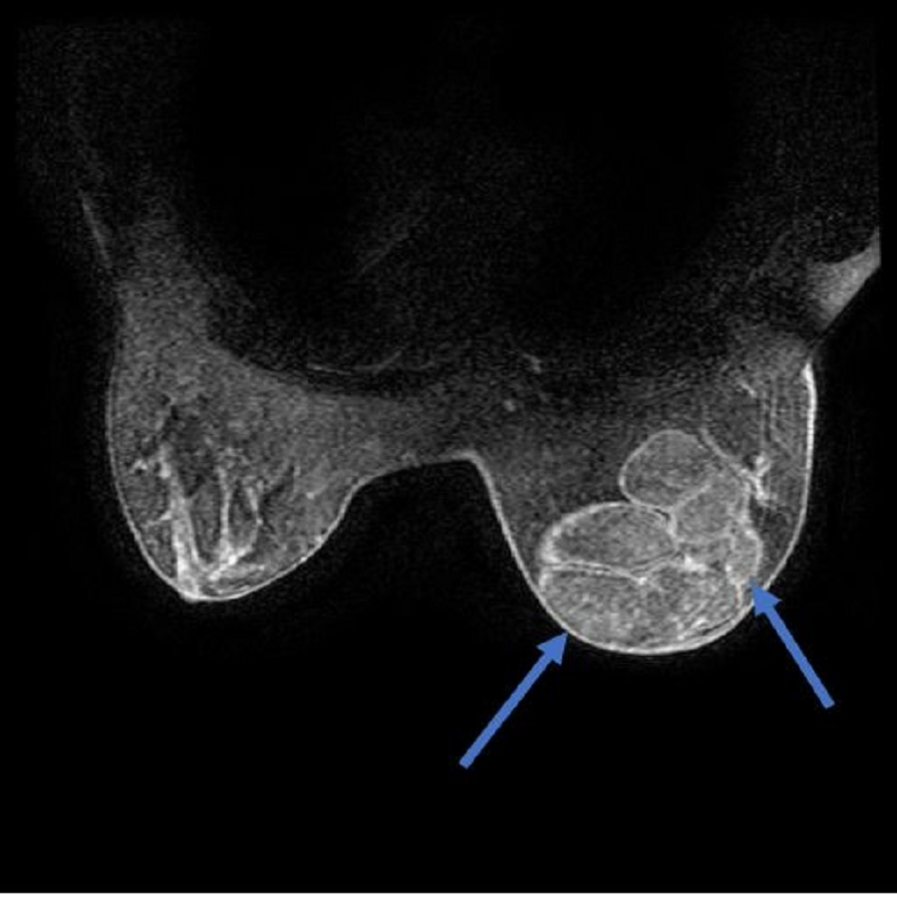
Magnetic resonance imaging of the mass
